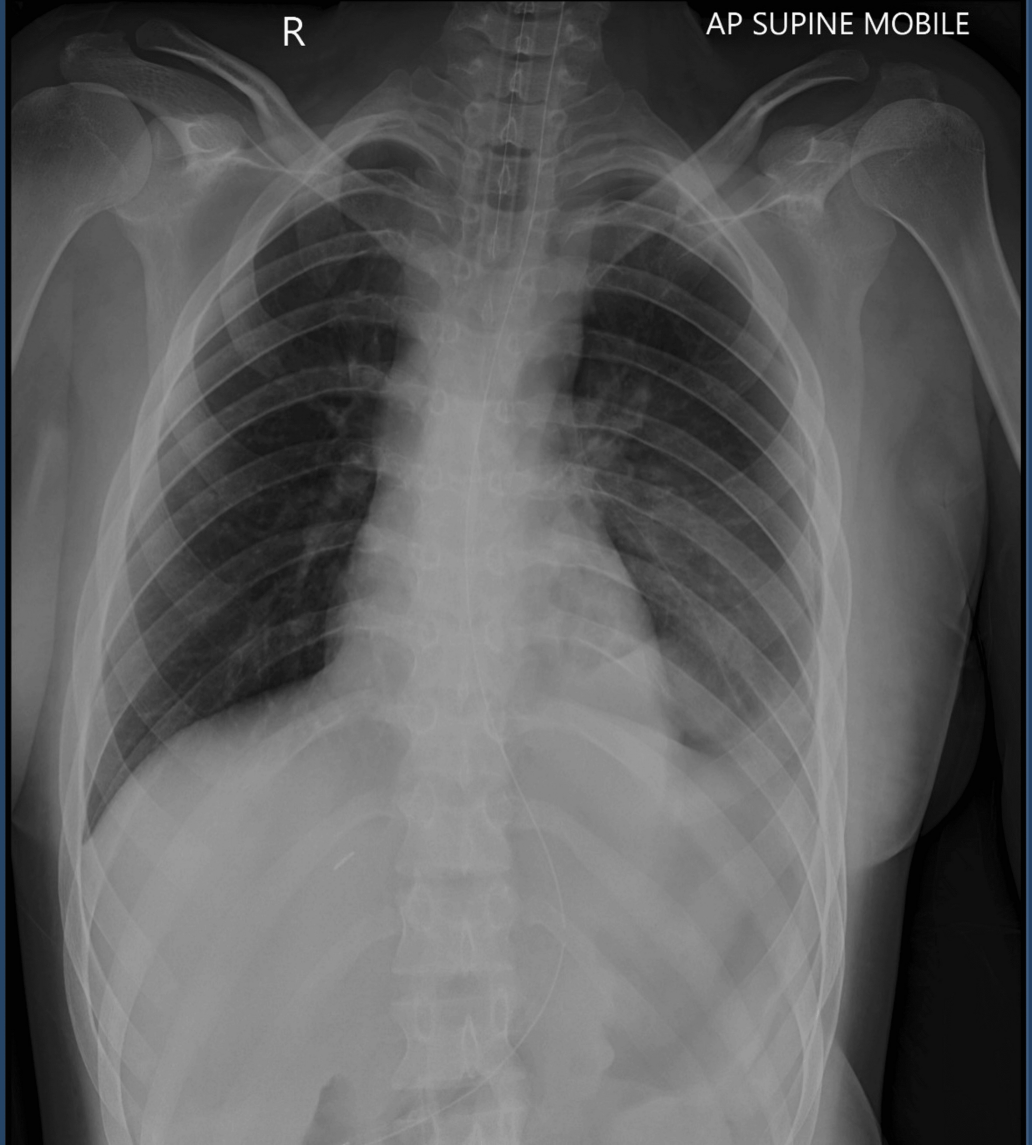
Postoperative chest radiograph (July 12, 2024) demonstrating satisfactory lung re-expansion with mild residual atelectasis and small postoperative pneumoperitoneum.
